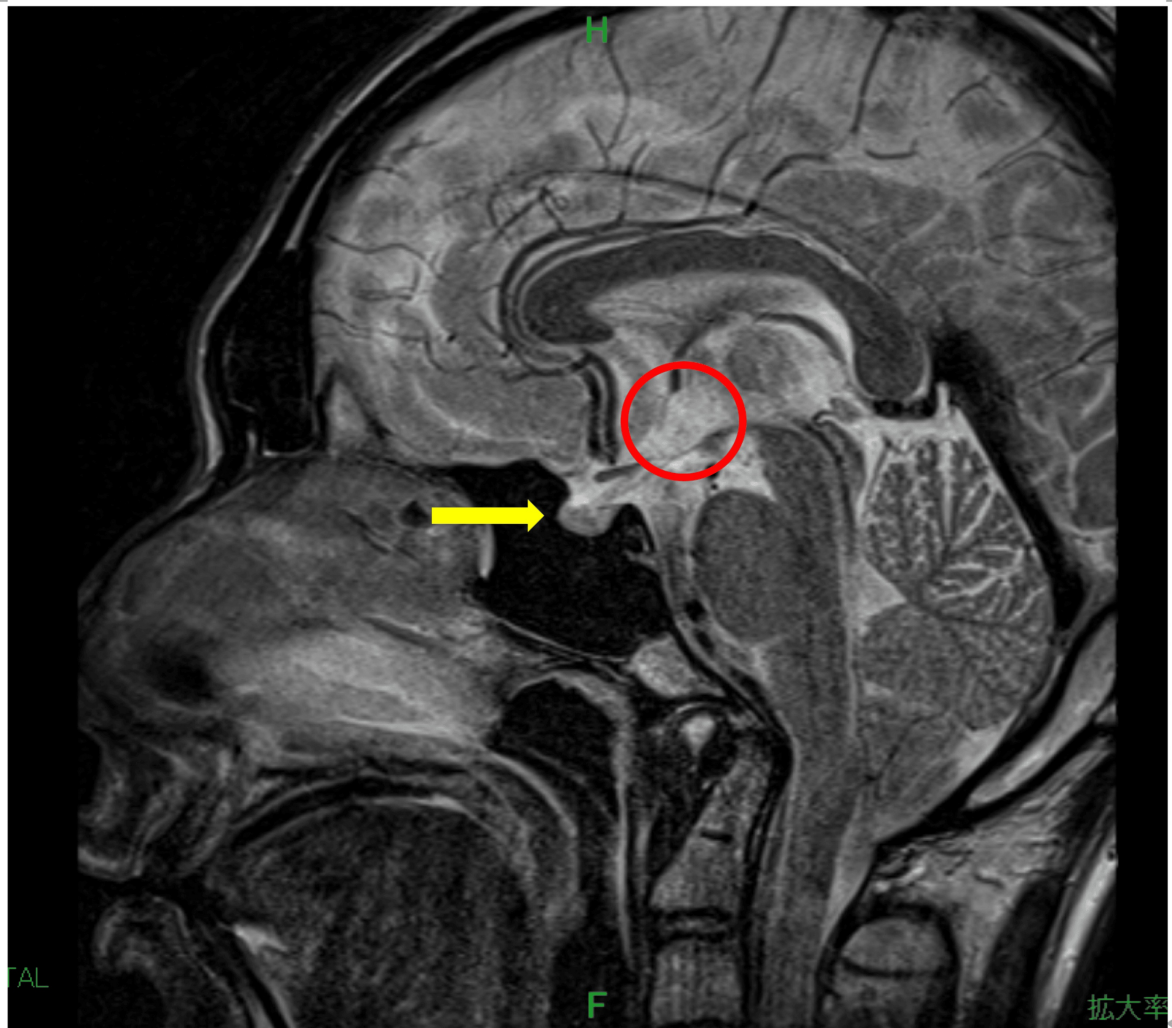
Sagittal T2-weighted MRI of the hypothalamus and pituitary gland on hospital day 1 The hypothalamus is indicated by a red circle, and the pituitary gland is marked with a yellow arrowhead.
